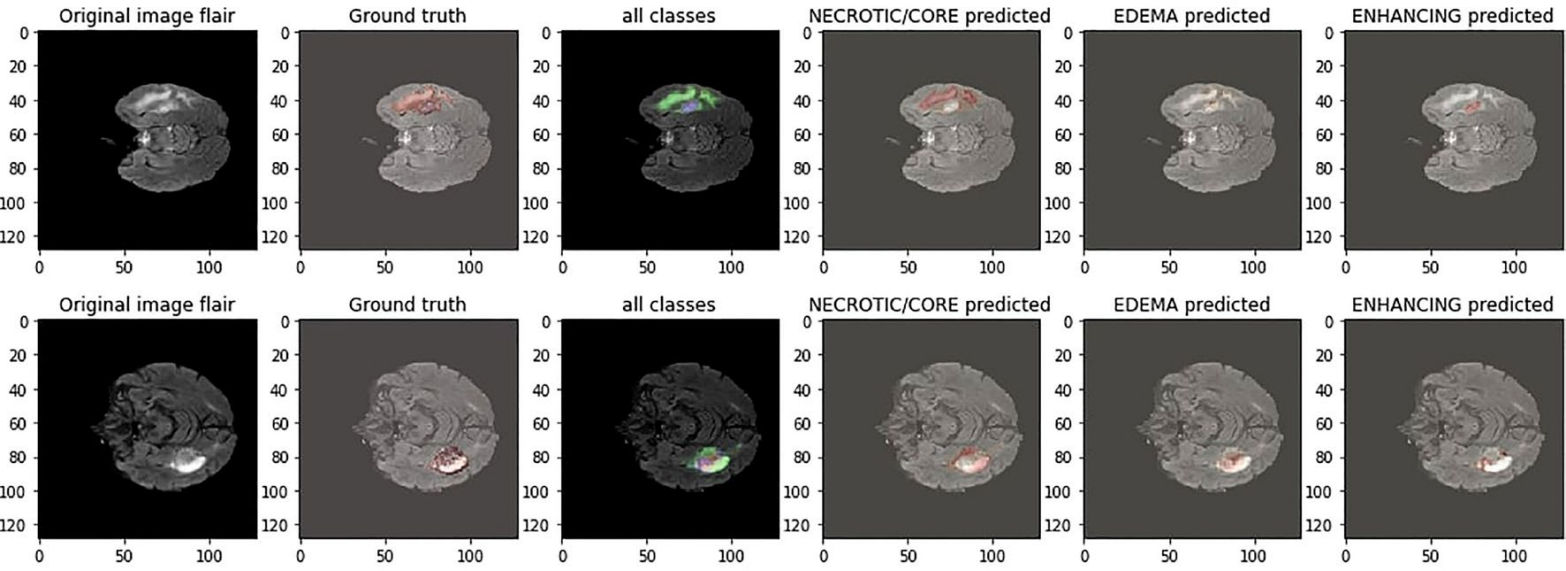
This image displays existing results, serving as a point of comparison with the visualization of qualitative results on BraTS2020 MRI sequences in^43^.
